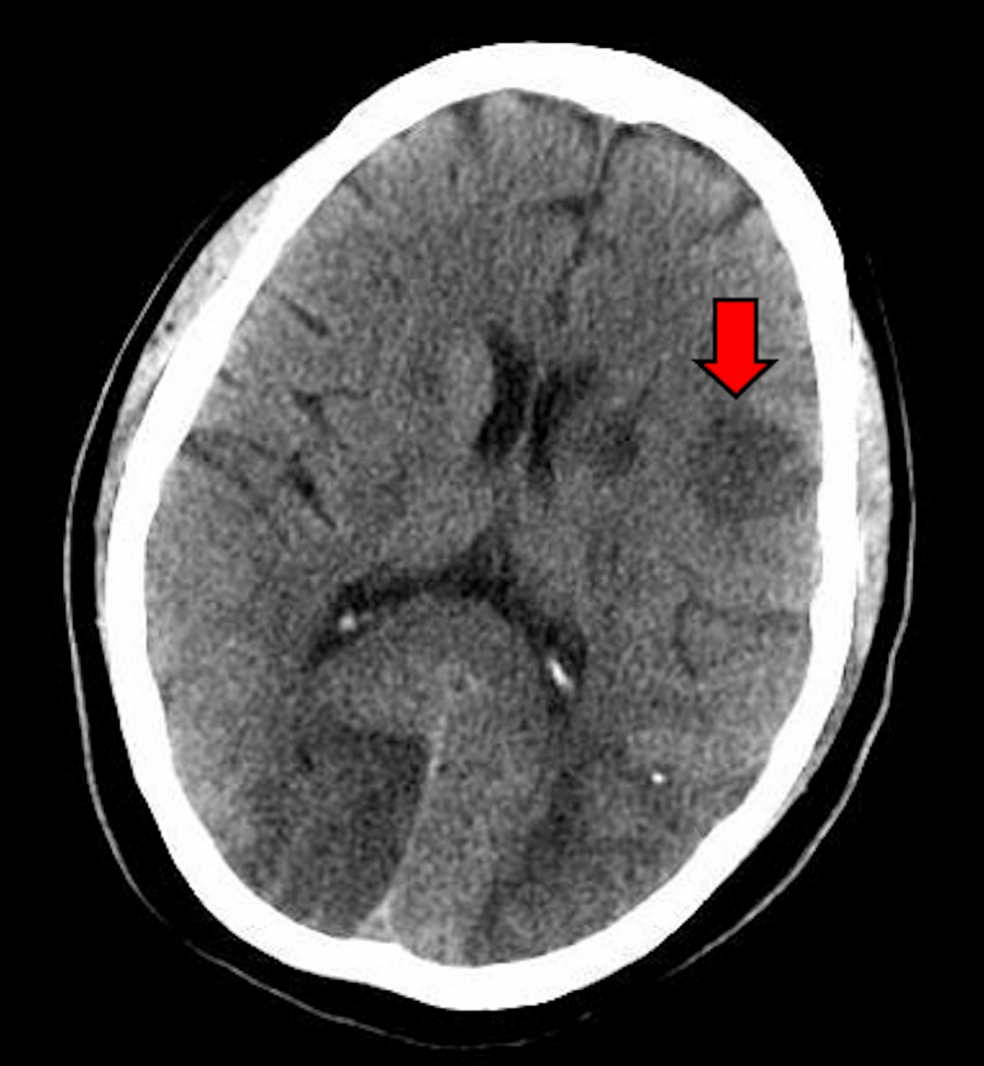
CT of the head demonstrating additional new infarcts during hospitalization (red arrow). CT: computed tomography
